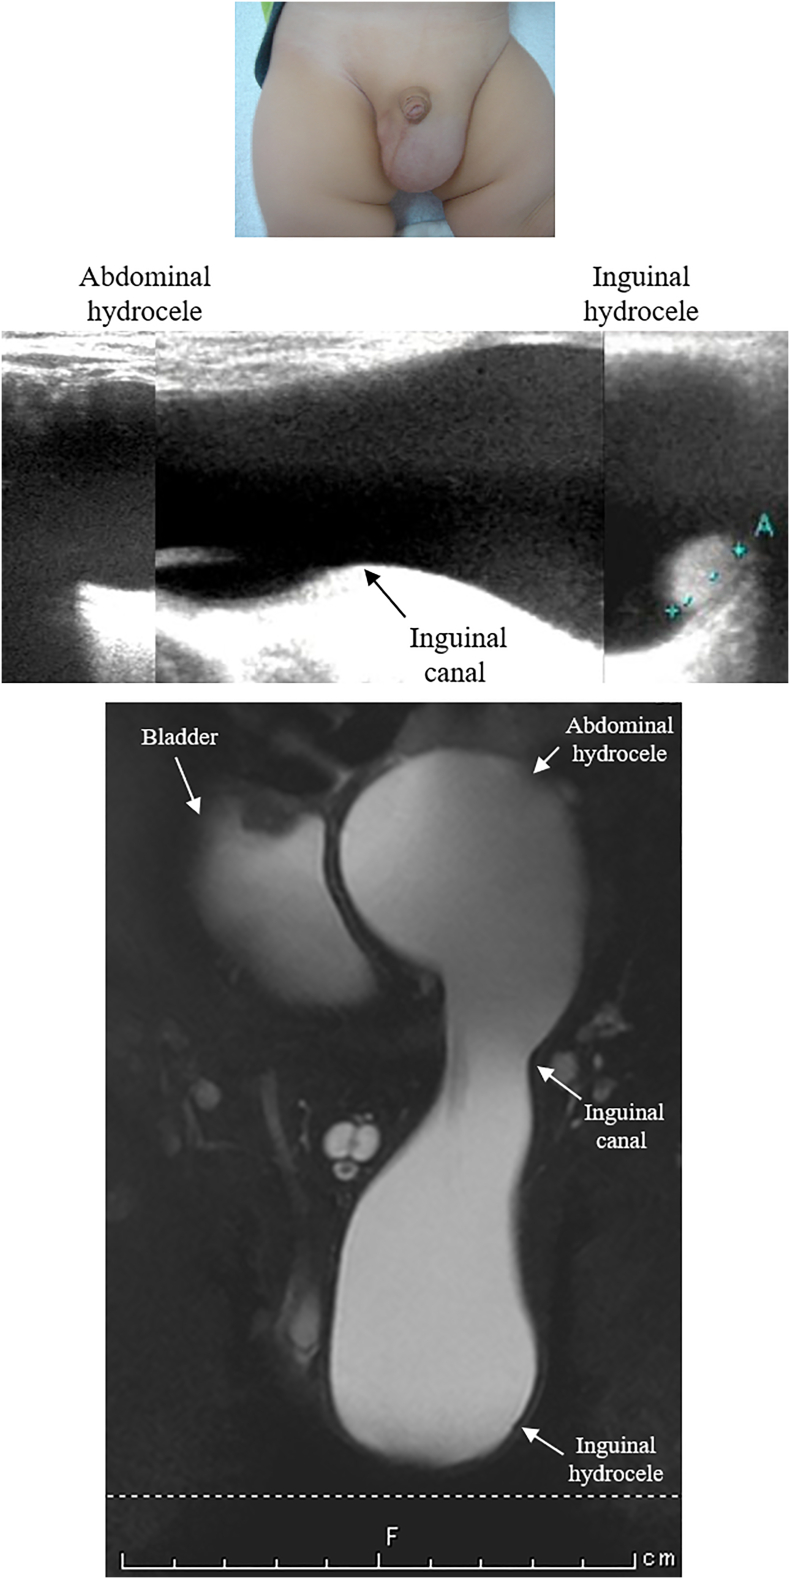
Abdominoscrotal hydrocele (ASH). (A) Development of ASH. (B) Ultrasonography findings. (C) Magnetic resonance imaging findings.
